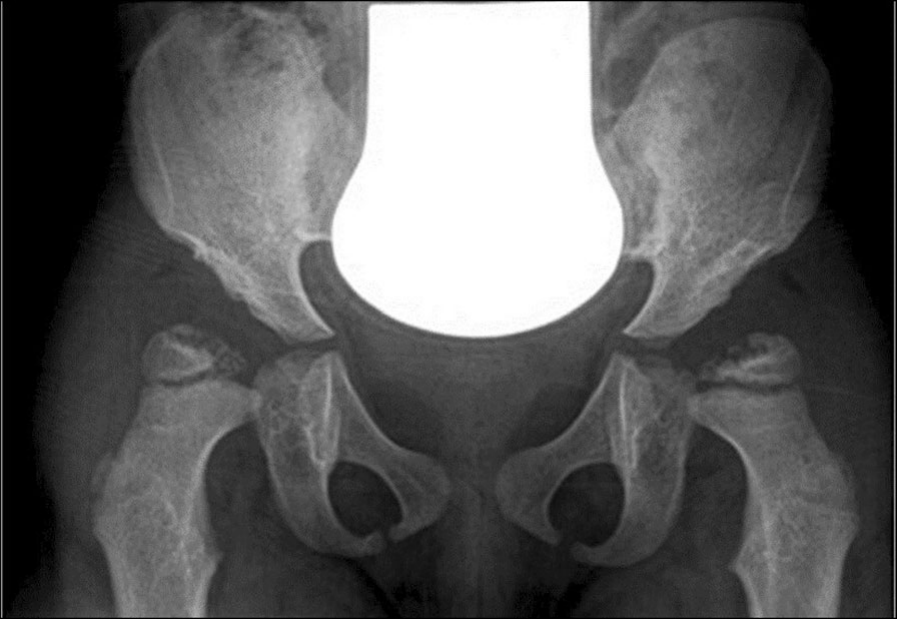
4-year-old with MPS VI. X-ray of the pelvis showing enlarged and receding acetabulum, underdeveloped femoral epiphysis, and acetabulum warped with coxa valga
